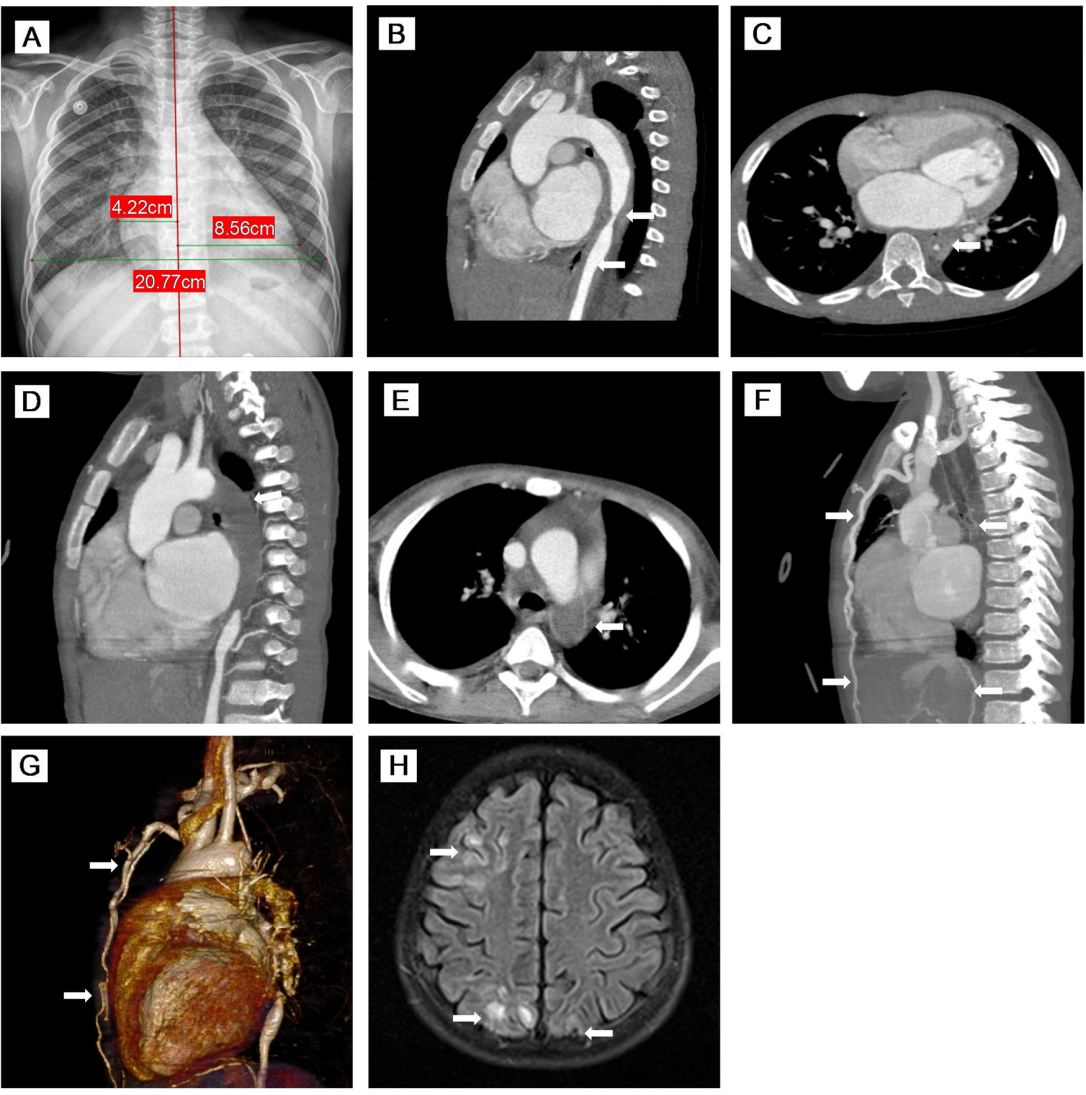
(A) Chest x-ray showed significant cardiomegaly. (B–E) MPR images of thoracic CTA. (B) Sagittal view of CTA demonstrated stenosis of the descending aorta and upper abdominal aorta with thickening of the vascular wall. (C) Axial view of CTA showed that the descending aorta was stenosed to 3.1 mm. (D,E) Sagittal oblique view and axial view of CTA indicated complete occlusion of the descending aorta with large thrombus (76 mm in length). (F,G) MIP image and VR reconstruction of CTA revealed complete occlusion of the descending aorta with large thrombus (76 mm in length) and extensive collateral circulation (twisted and dilated right intrathoracic artery, numerous open collateral vessels in the neck and upper mediastinum). (H) Axial view of brain MR imaging revealed high signals on T2 FLAIR images of cortical and subcortical areas of both the occipital lobes and right frontal-parietal lobe. MPR, multiplanar reconstruction; CTA, computed tomography angiography; MIP, maximum intensity projection; VR, volume rendered; MR, magnetic resonance; FLAIR, fluid-attenuation inversion recovery.
